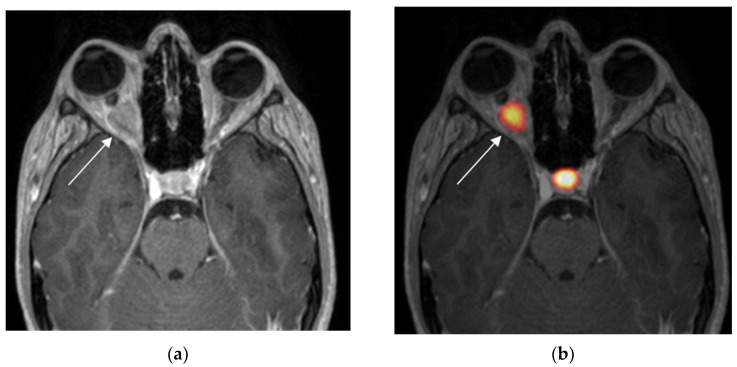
Case 1. Axial MRI T1WI (a) showing outer enhancement of a right intraorbital tumour and axial fused MRI, and ^68^Ga-DOTATOC PET images (b) showing intense tracer uptake in the right intraorbital tumour (white arrow) and intense physiologic uptake in the pituitary: tumour SUV_max_ = 11.9; pituitary SUV_max_ = 17.
